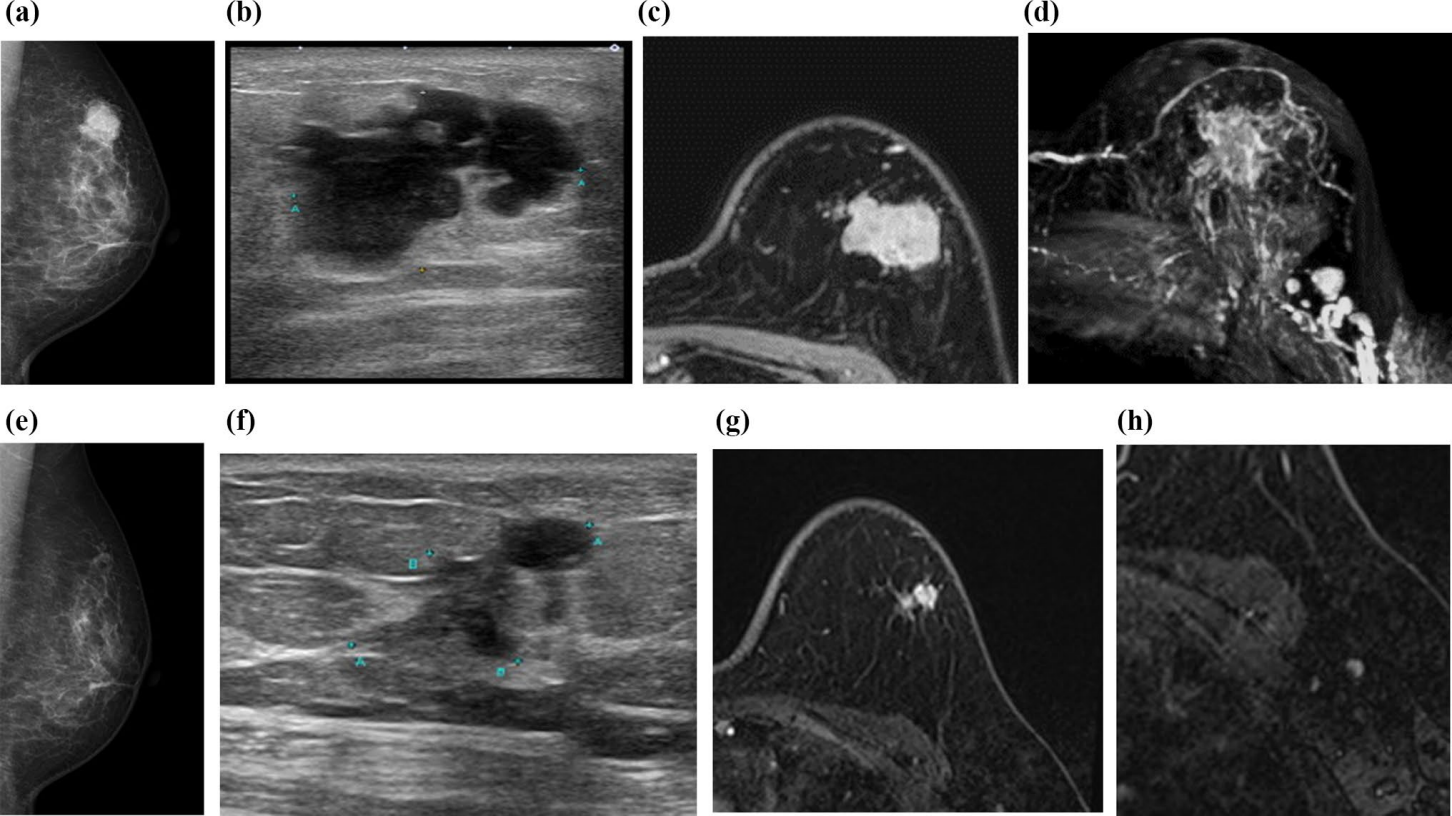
Pre- and post-treatment imaging of a woman in her 50s with ER-positive, HER2-negative breast cancer (Case 1). a–d Pretreatment imaging: a Mammography of the left breast, b Ultrasonography of the left breast, c Initial phase of dynamic contrast-enhanced (DCE) MRI, d MIP image of DCE MRI. A patient in her 50 s presented with a 3-cm tumor in the left breast and six metastatic lymph nodes. Mammography revealed an irregularly shaped, high-density mass with microlobulated margins in the left upper quadrant (a). Ultrasonography demonstrated a hypoechoic lobulated mass without posterior enhancement (b). The initial phase of DCE MRI showed an irregularly shaped mass with rapid homogeneous enhancement and metastatic lymphadenopathy in the left axilla (c, d). These findings were consistent with axillary lymph node metastasis involving four or more nodes, thereby meeting the eligibility criteria of the MonarchE trial. e–h Post-treatment imaging following neoadjuvant chemotherapy (FEC and DTX): e Mammography of the left breast, f Ultrasonography of the left breast, g Initial phase of DCE MRI, h Delayed phase of DCE MRI. Post-neoadjuvant chemotherapy imaging revealed significant tumor shrinkage and no residual lymphadenopathy (e–h). The scans demonstrated a complete or near-complete response, leading to continuation of CDK4/6 inhibition in the adjuvant setting (post-surgery) and resulting in long-term disease control
